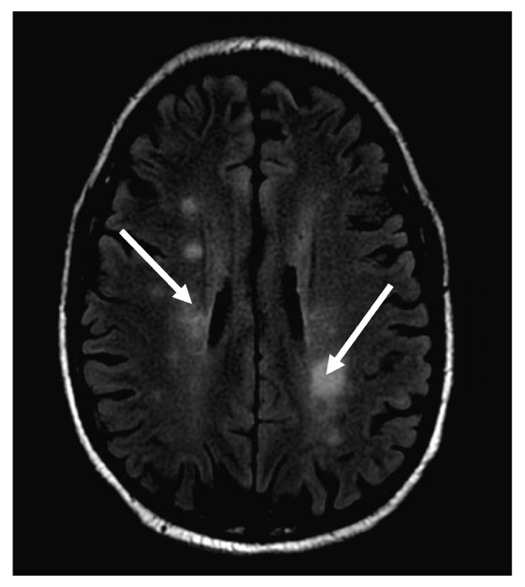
Characteristic T1-weighted MRI brain findings of Bing Neel Syndrome (BNS) where lymphoplasmacytic cells invade the CNS. The white arrows indicate areas of enhancing periventricular white matter foci that occur in BNS
